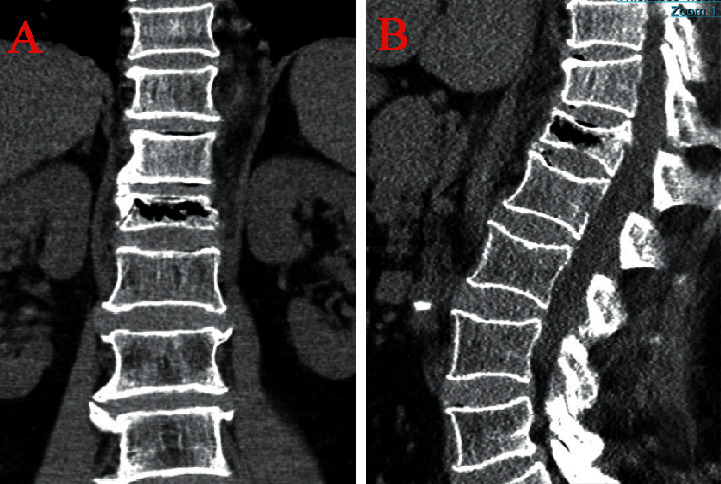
Intravertebral vacuum cleft was visible on coronal (a) and sagittal (b) views of computed tomography.
